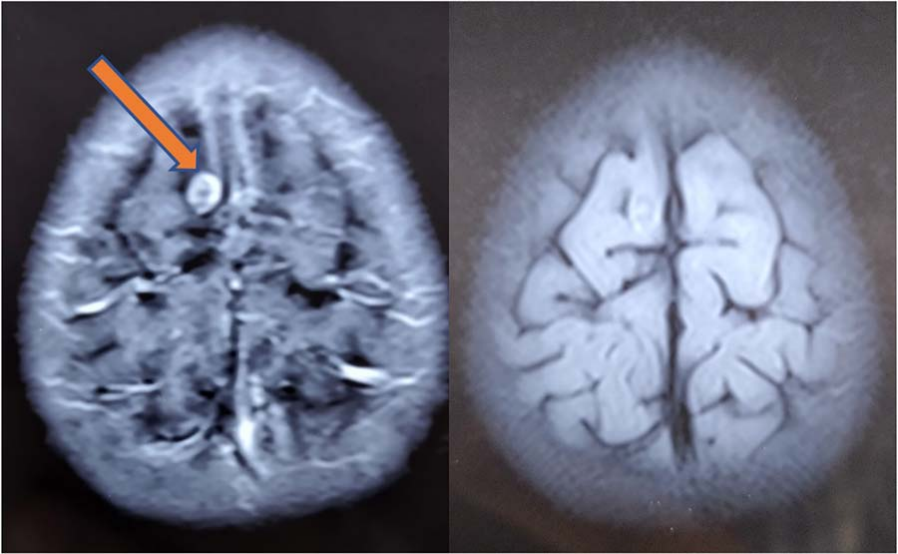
Repeat MRI at 6 months showing resolved changes with reduction in size of the lesion with resolution of perilesional edema.
